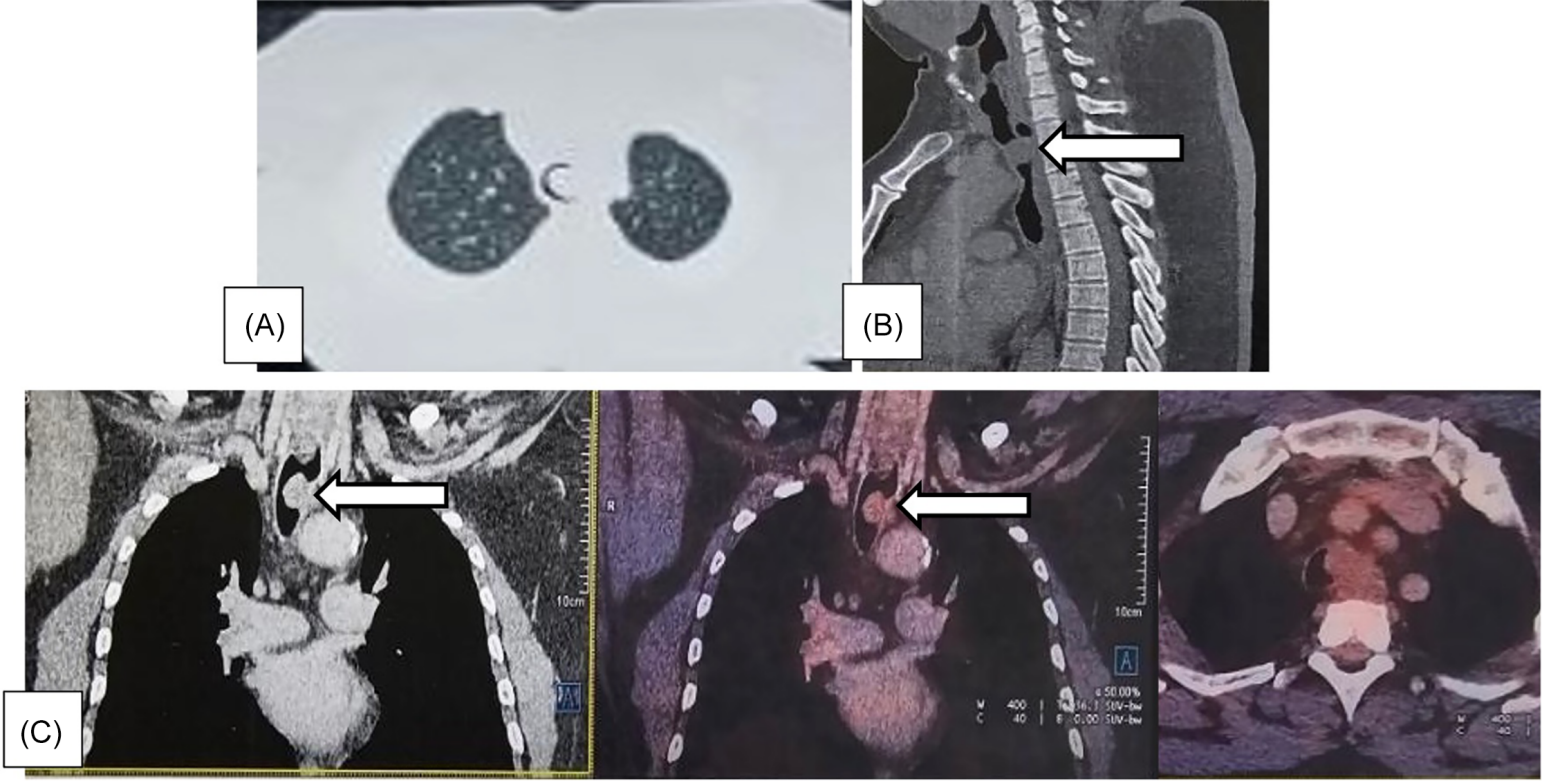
Chest computerized tomography and positron emission tomography‐CT. (A). Axial CT image and (B) sagittal view showing the tumour inside the lumen of the trachea arising from the left lateral wall (arrow). (C) PET‐CT scan image showing low grade FDG avid soft tissue mass with intraluminal component/occlusion noted involving the trachea at the level of manubrium sternum (arrow).
